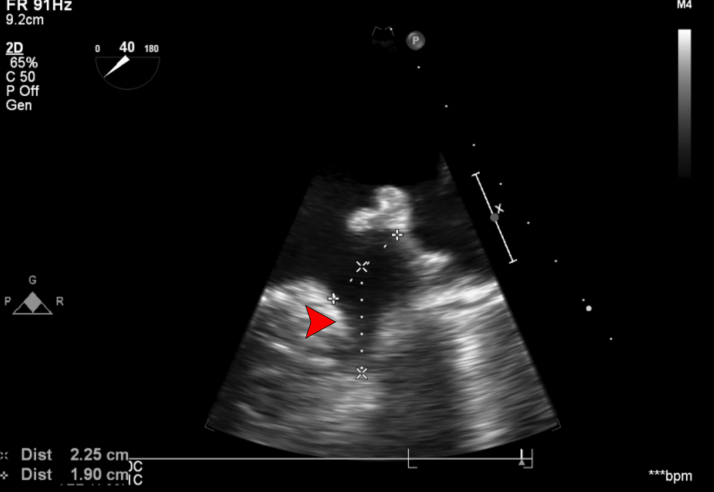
TEE Midesophageal Short-Axis View at 40° Showing the Ostial Diameter of 1.90 cm (+) and Depth of 2.25 cm (×) The red arrowhead indicates the left atrial appendage. TEE = transesophageal echocardiography.
